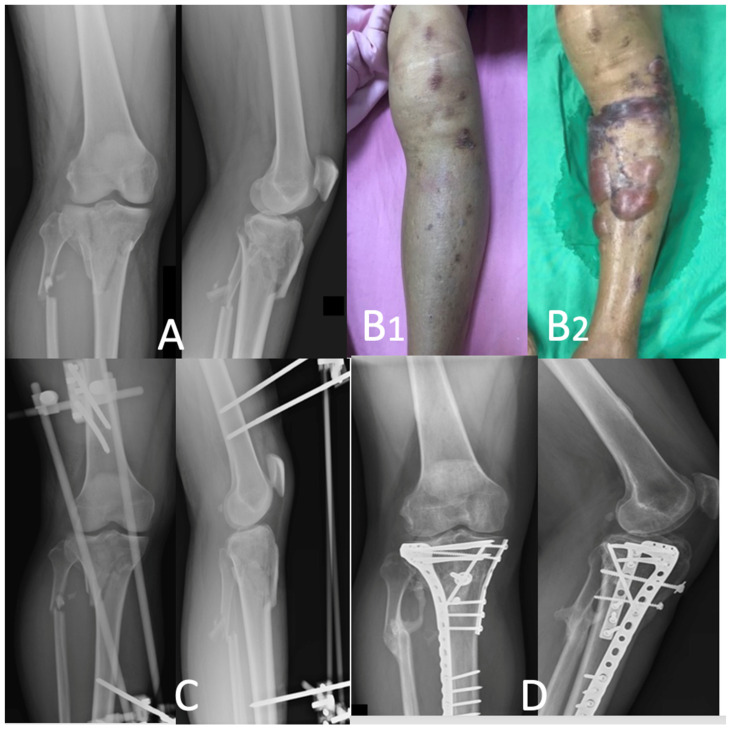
Typical case of bicondylar tibial plateau fracture with soft tissue swelling. A staged operation from external fixation to internal fixation is shown (A). A 44-year-old male sustained a Schatzker type VI tibial fracture. Plain films of comminuted proximal tibia fractures are demonstrated. Soft tissue swelling upon presentation is shown in (B1), and considerable bullous formation for suspicious compartment syndrome is shown in (B2). (C) Immediate stabilization with external fixation, waiting for the subsidence of soft tissue swelling, is performed (D). Definite fixation is completed with double plating 7 days after external fixation, with good healing of the fracture.
